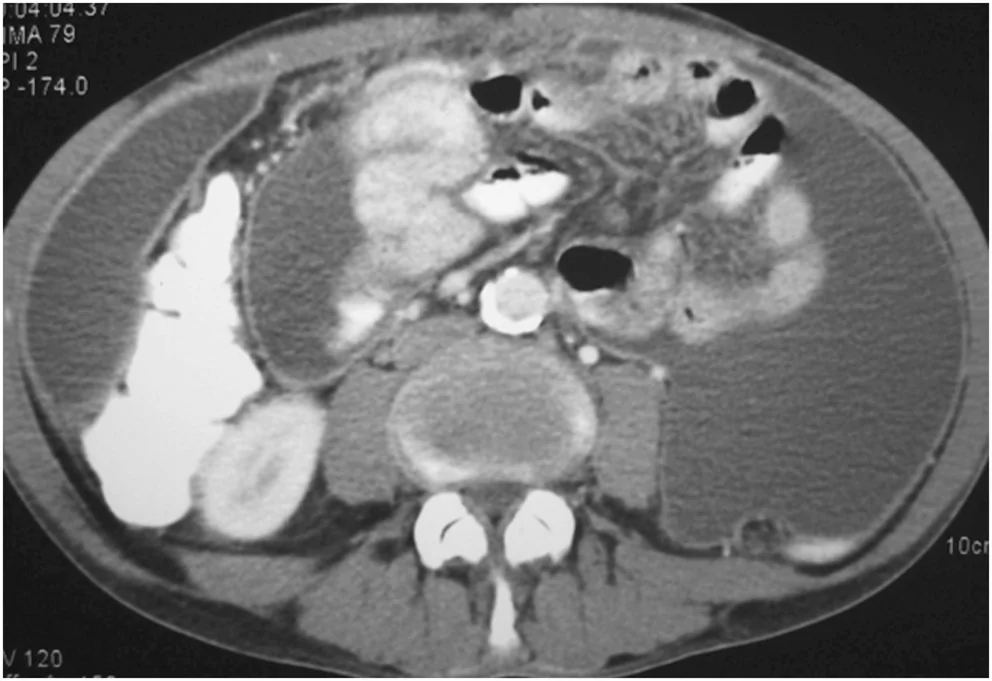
Abdominal computed tomography showed septate ascitic fluid in various locations, with significant thickening of the parietal peritoneum.
